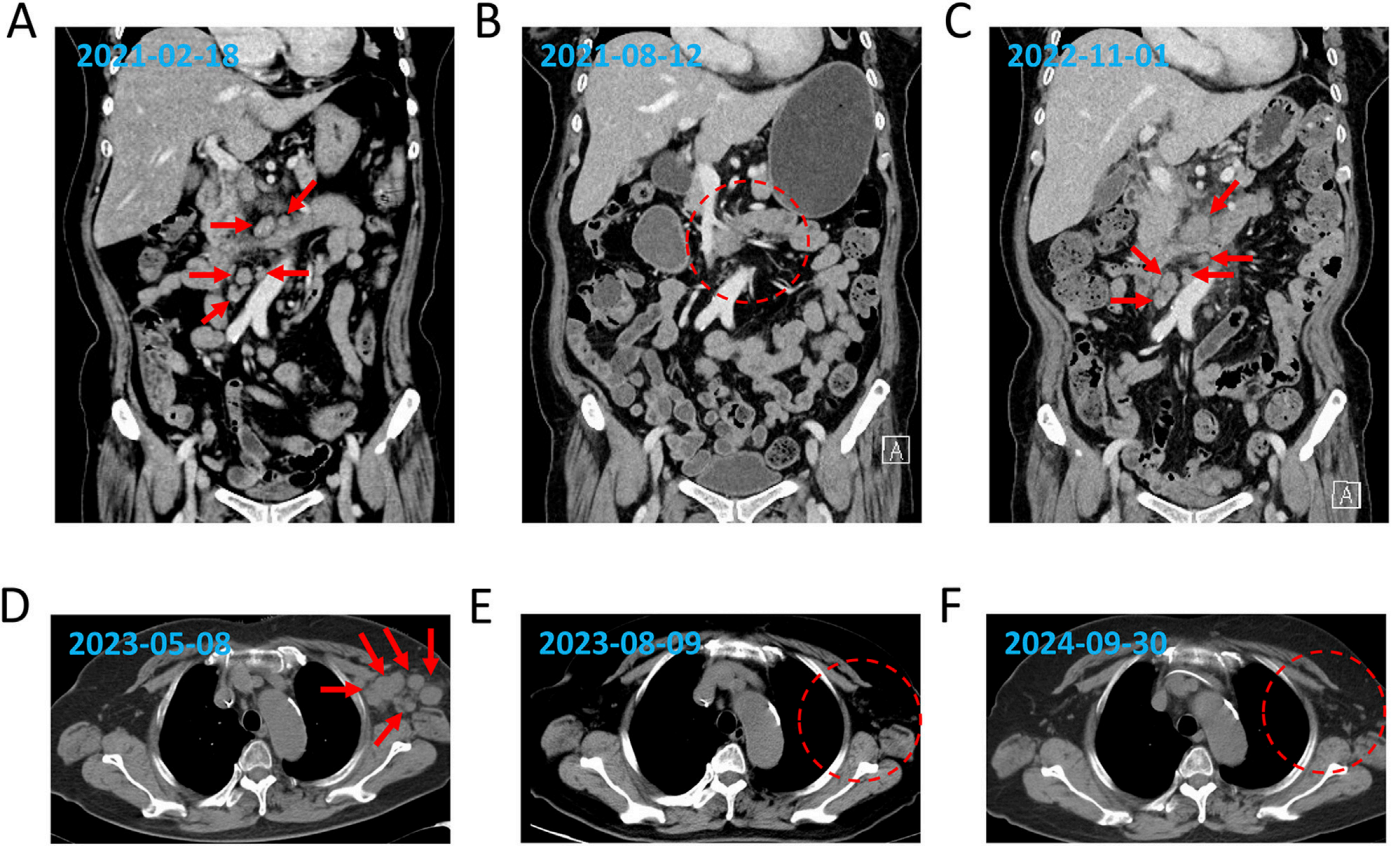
Dynamic evaluation of enlarged lymph nodes using CT. Enlargement and reduction of lymph nodes at initial diagnosis (A), PR1 (B), relapse (C), refractory (D), PR2 (E), and SD (F) by CT evaluation. The red arrows indicate the enlarged lymph nodes, and the red dotted frames represent the reduction of the enlarged lymph nodes.
